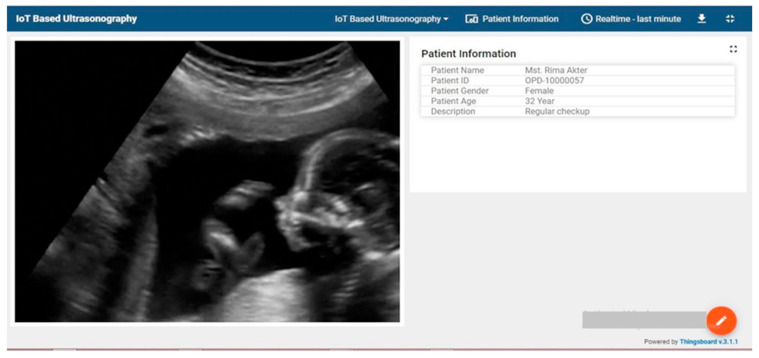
Ultrasound image view with patient information.
